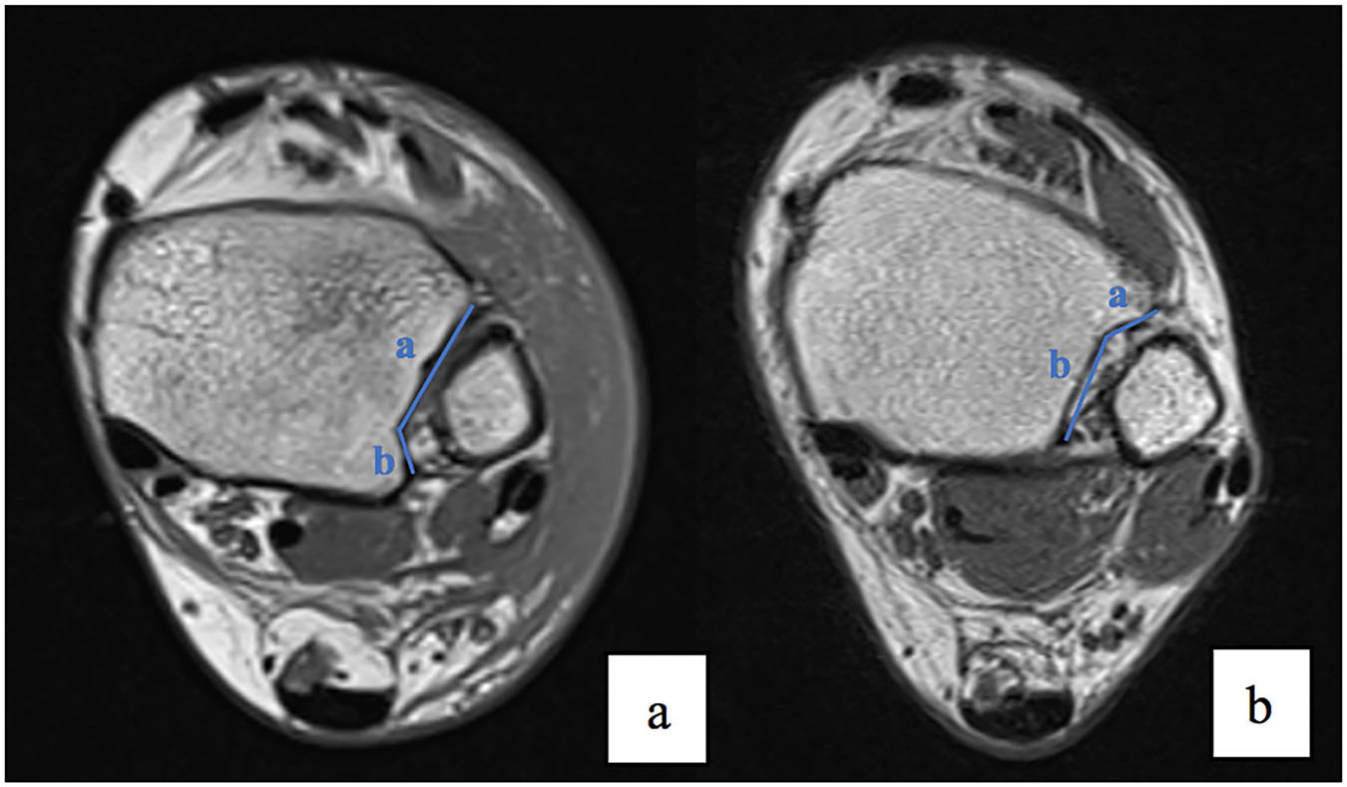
MRI T1-weighted images on the axial plane, at the same level, 1 cm above the tibio-talar joint of two different patients. Note the different morphology of the DTS. a A patient with a posterior position of the maximum measurable depth of the fibular notch, with a calculated *a*/*b* ratio of 2.43. b A patient with a lower *a*/*b* ratio of 0.61, with a smaller anterior facet length of the fibular notch when compared with the posterior facet length, due to a more prominent anterior tibial tubercle
